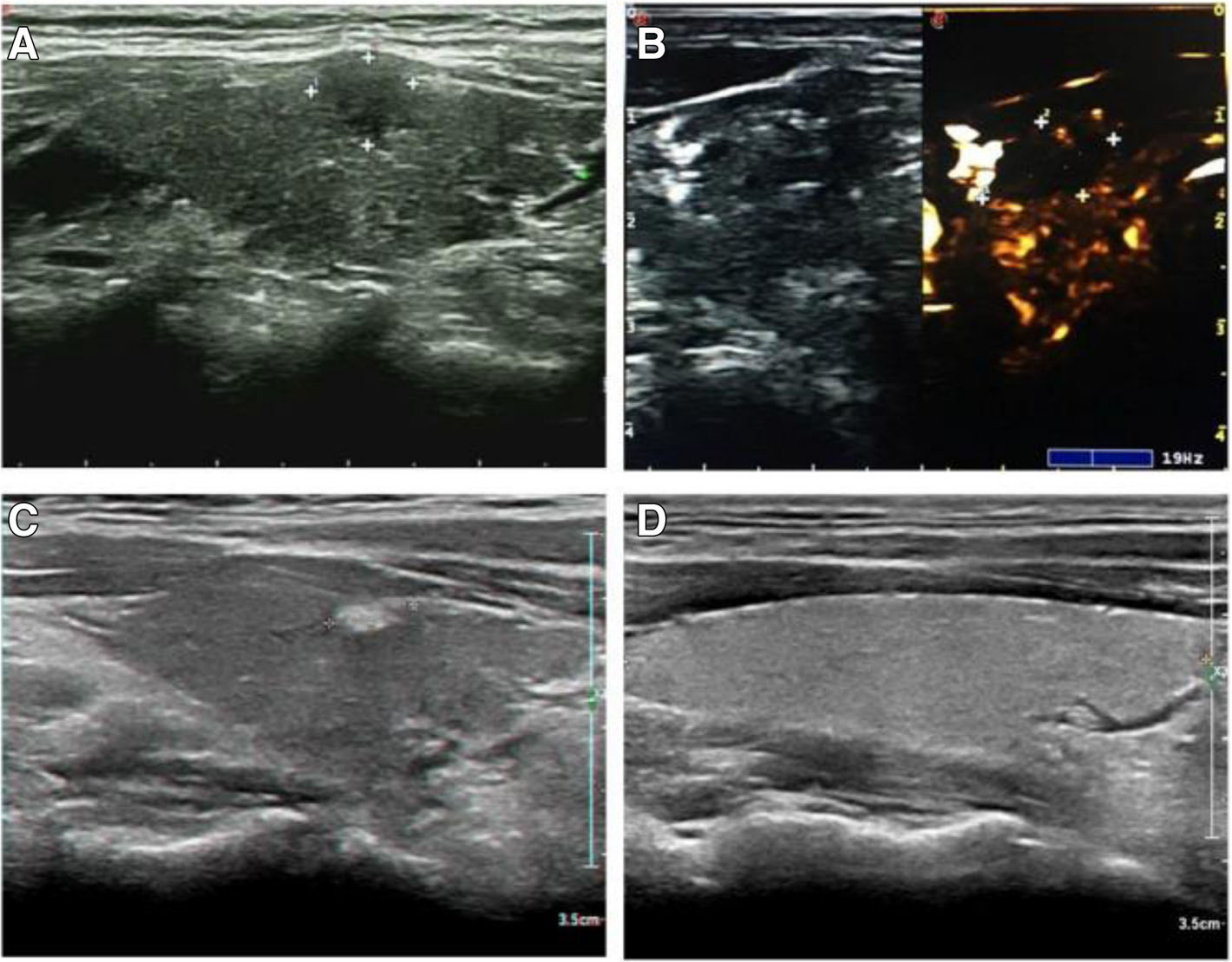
a 47-year-old male with a PTMC lesion in whom conventional ultrasound examination showed a 7.8 mm × 6.8 mm × 4.3 mm hypoechoic nodule. b After PLA, CEUS revealed a hypoechoic area in the original area, along with some debris in the centre that presented as strong echoes, which demonstrated that the lesion had been completely ablated. c Six months after PLA, conventional ultrasound examination showed a 7.1 mm × 3.2 mm × 2.1 mm hyperechoic nodule. d Eighteen months after PLA, conventional ultrasound examination showed that the lesion had completely disappeared
